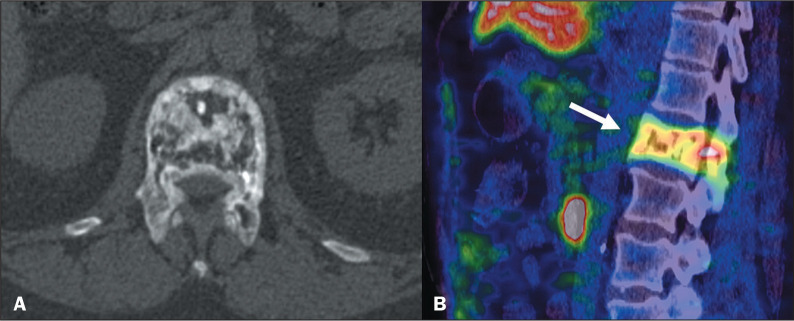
Preoperative ^68^Ga-PSMA PET/CT of a 70-year-old male
